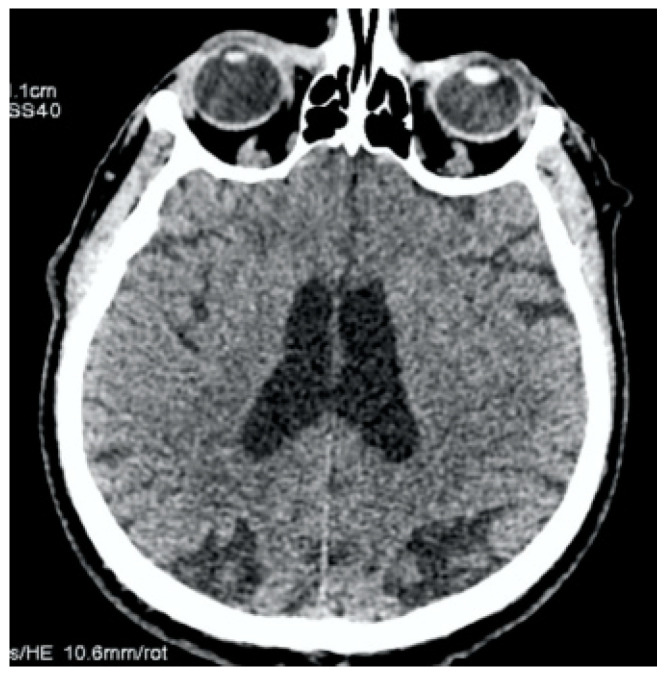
Clinical Case 1, Patient M., 73 years old. Brain MSCT in 20 min after symptoms’ onset. No data for ischemia or brain damage; ASPECTS = 10.
